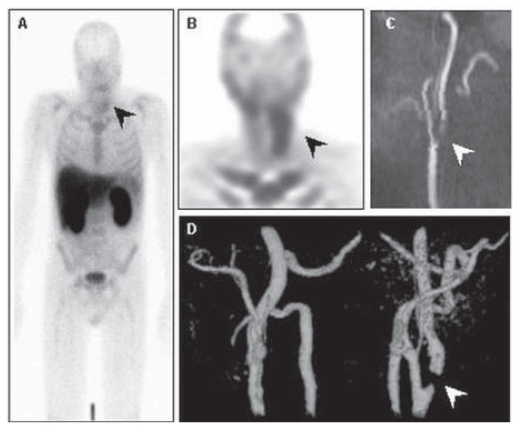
Planar (A) and Annexin V SPECT (B) images show pathological tracer uptake in the left side of the neck (black arrows), corresponding with prominent stenosis of the left carotid artery (white arrows) in the sagital (C) and frontal (D) MIP from MR angiography.
